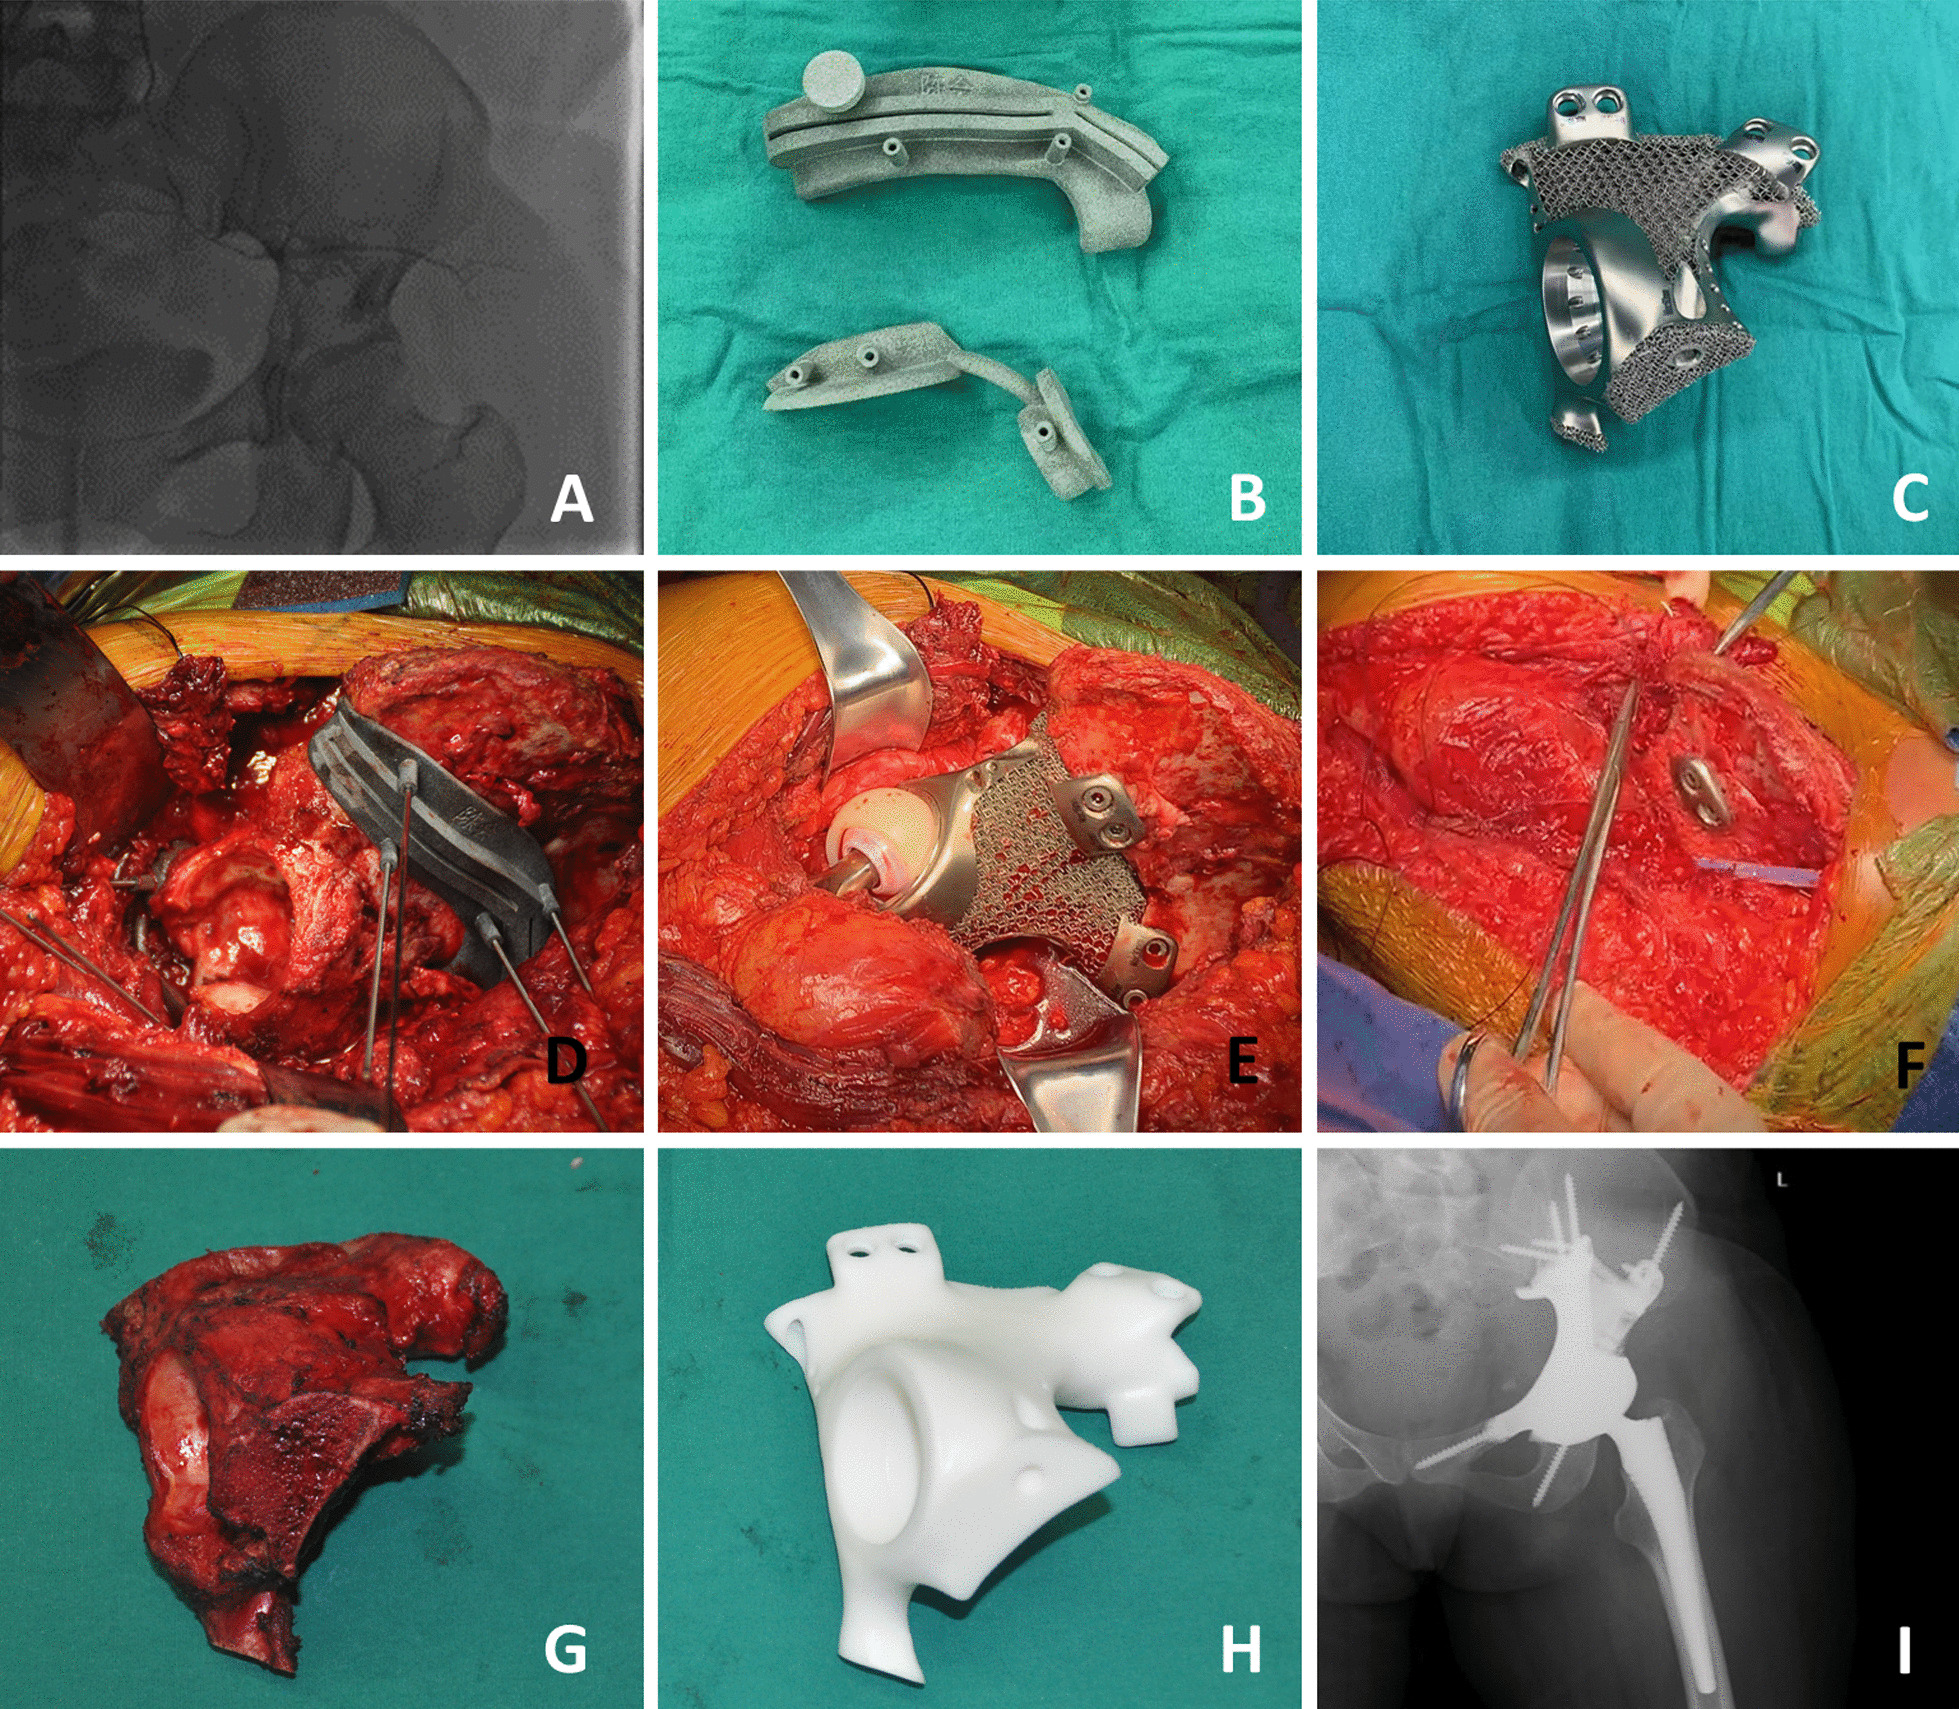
Surgical resection and implantation procedure. A Preoperative tumor vascular embolization. B Customized osteotomy template. C 3D-printed hemipelvic prosthesis. D Intraoperative osteotomy performed with the aid of the customized osteotomy template. E Implantation of the 3D-printed hemipelvic prosthesis. The reconstructed joint did not dislocate even in an extreme position. F Suturing of the surrounding soft tissue. G, H Comparison of the intact hemipelvic bone and the 3D-printed hemipelvic prosthesis. I Radiograph obtained 6 months after the procedure
